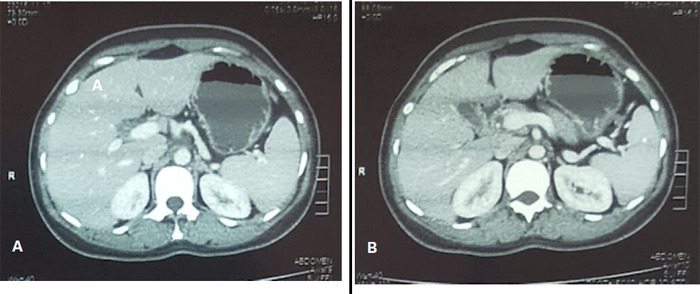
Abdominal CT scan reveals an absence of the body and tail of the pancreas.
